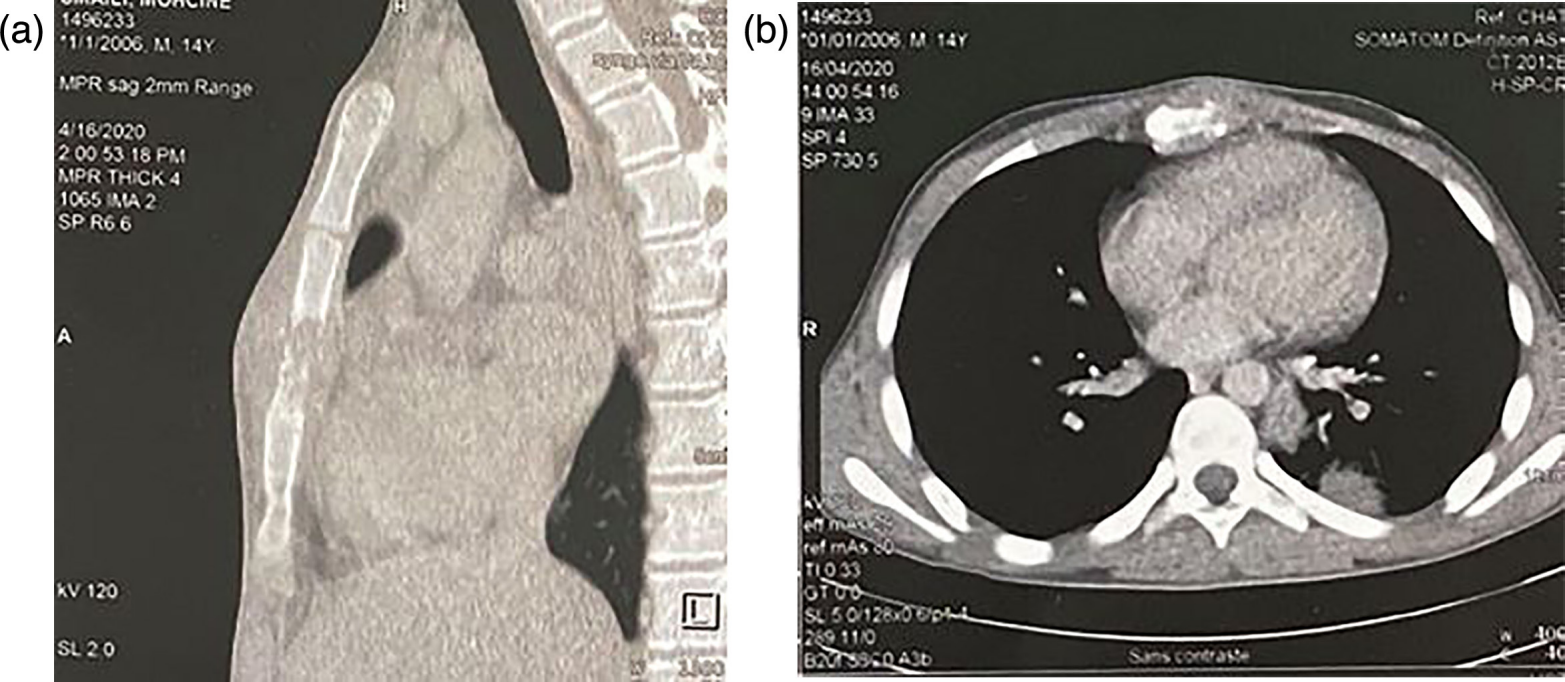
Chest CT scan showing an osteolytic tissue mass centred on the sternum (left, axial section; right, sagittal section).
